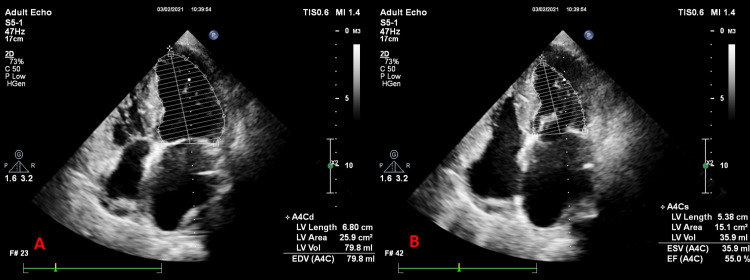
Ejection fraction calculation by Simpson’s method. (A) Apical four-chamber view in diastole (A4Cd). (B) Apical four-chamber view in systole (A4Cs). EF: ejection fraction; LV: left ventricle
